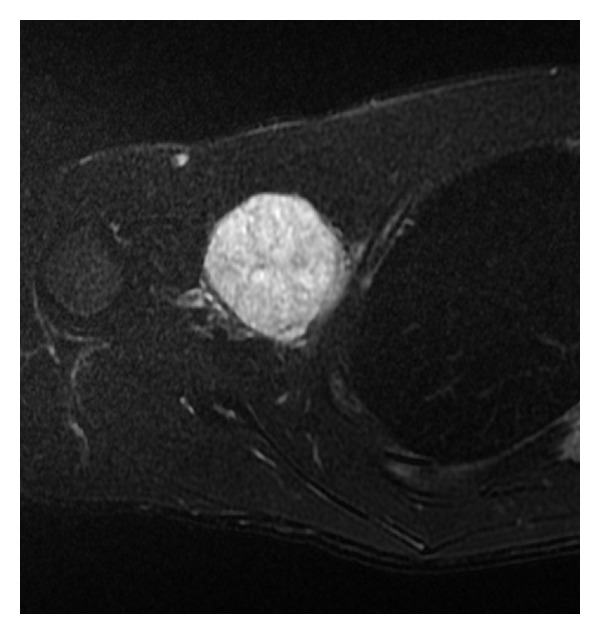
Axial fat-suppressed fast-spin echo T2-weighted (8500/63) image of the right axilla shows homogeneous increased signal intensity throughout the lesion. There is no surrounding soft tissue edema pattern and the adjacent bone marrow signal is normal.
